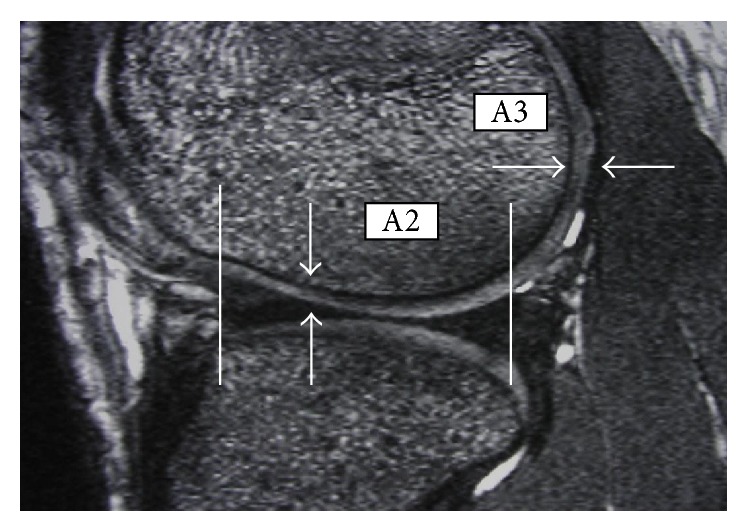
Mapping of MRI showing regions that are A2 and A3. White arrows show thickness in these 2 regions.
